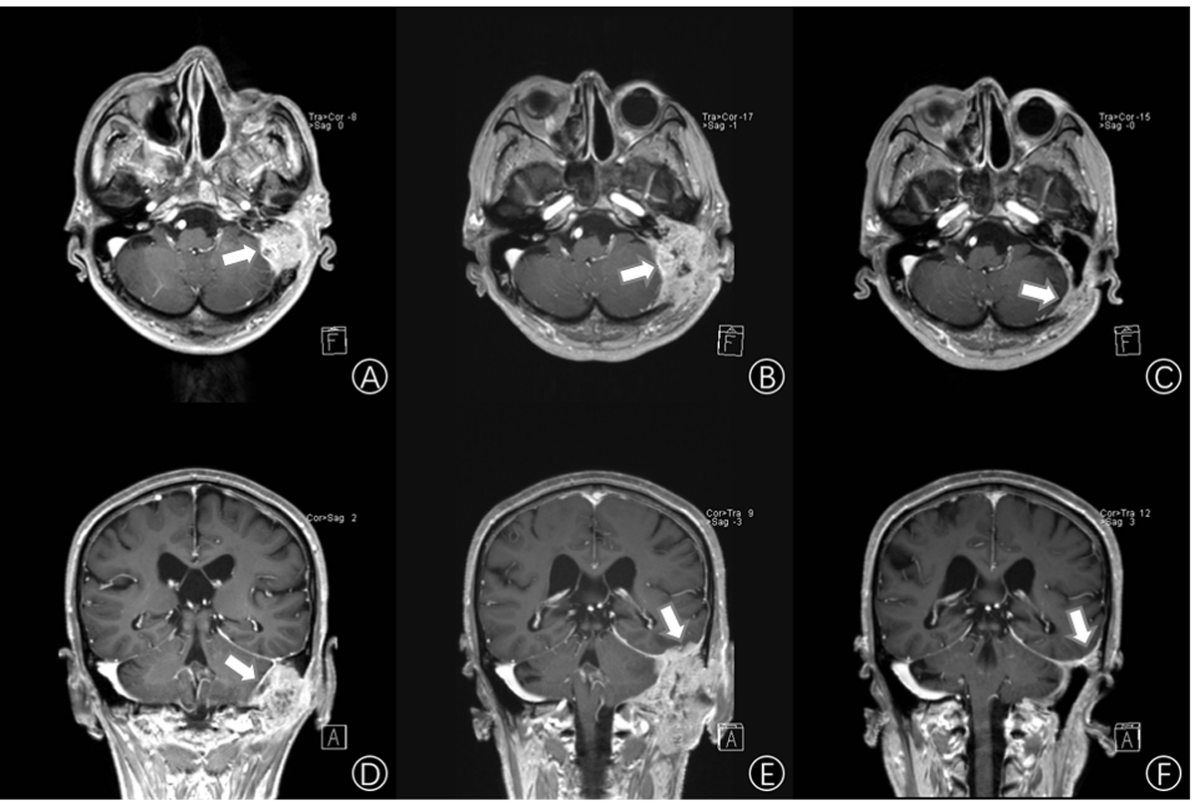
Imaging examination A. and D. Enhancement cerebral MRI showing the left temporal lobe space-occupying lesion (white arrows) (size:27.5mm x 38.0mm x 40.5mm). B. and E. Three months after the first middle ear surgery, enhancement cerebral MRI shows the tumor (white arrows) is recurrent (size:40.5mm x 52.3mm x 63.6mm). C. and F. Two weeks after bevacizumab and pemetrexed treatment, an enhancement cerebral MRI shows that the right temporal lobe space occupying lesion has reduced by 95% (size: 13.2mm x 20.5mm x 27.0mm). (A, B, C. Horizontal enhancement imaging. D, E, F. Coronal enhancement imaging).
